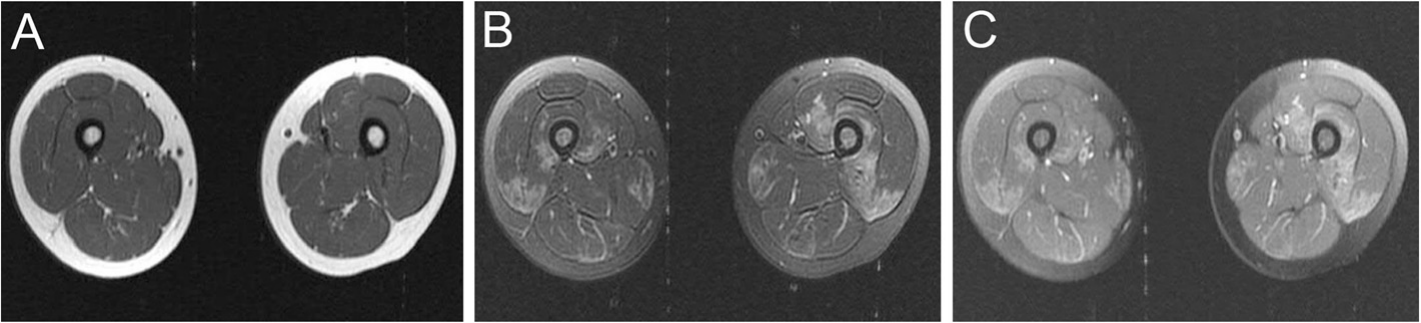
MRI of the thigh musculature demonstrating enhancement consistent with myopathy. (A) T1, (B) T2 FSE, and (C) fat saturated T1 post gadolinium images demonstrate normal T1 signal and patchy increased T2 signal with corresponding gadolinium enhancement in the vastus intermedius, vastus lateralis muscles.
